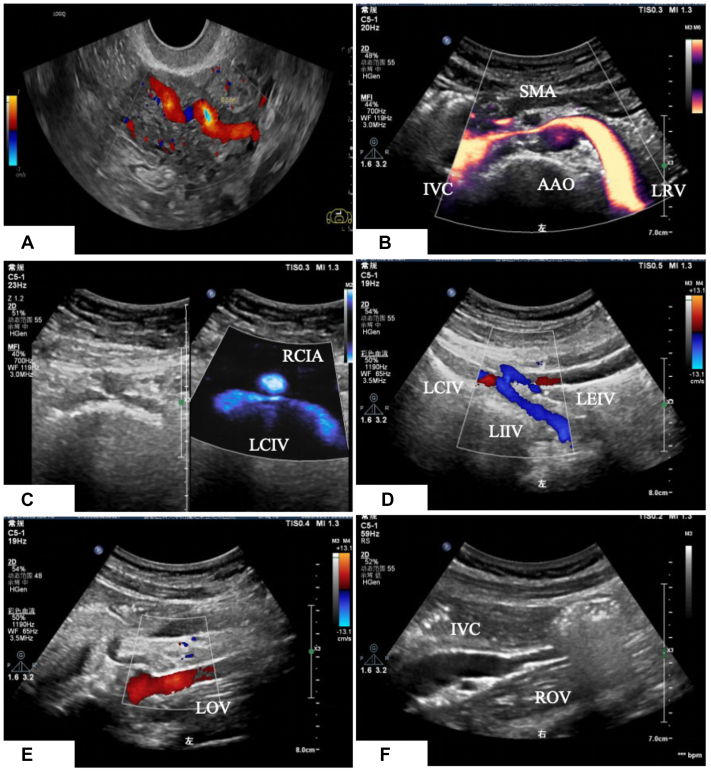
Ultrasound images of the abdominopelvic vessels. (A) Arc-shaped uterine veins with a left-to-right shunt. (B) Nutcracker phenomenon. (C) Left common iliac vein compression. (D) Left internal iliac vein reflux. (E) LOV reflux. (F) ROV. *AAO*, abdominal aorta; *IVC*, inferior vena cava; *LEIV*, Left external iliac vein; *LIIV*, left internal iliac vein; *LOV*, left ovarian vein; *LRV*, left renal vein; *RCIA*, right common iliac artery; *ROV*, right ovarian vein; *SMA*, superior mesenteric artery.
